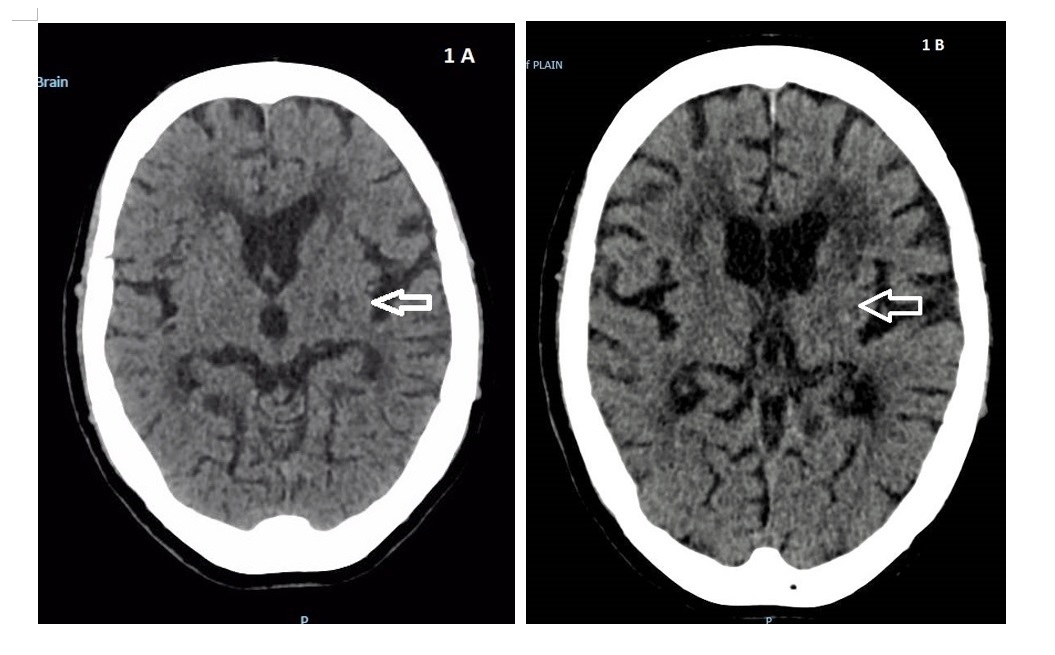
Computed Tomography (CT) of the Brain 1A (left): CT brain on first presentation showing hypodensity in the left corona radiata. 1B (right): CT brain near three months later showing hyperdensity in the left corona
